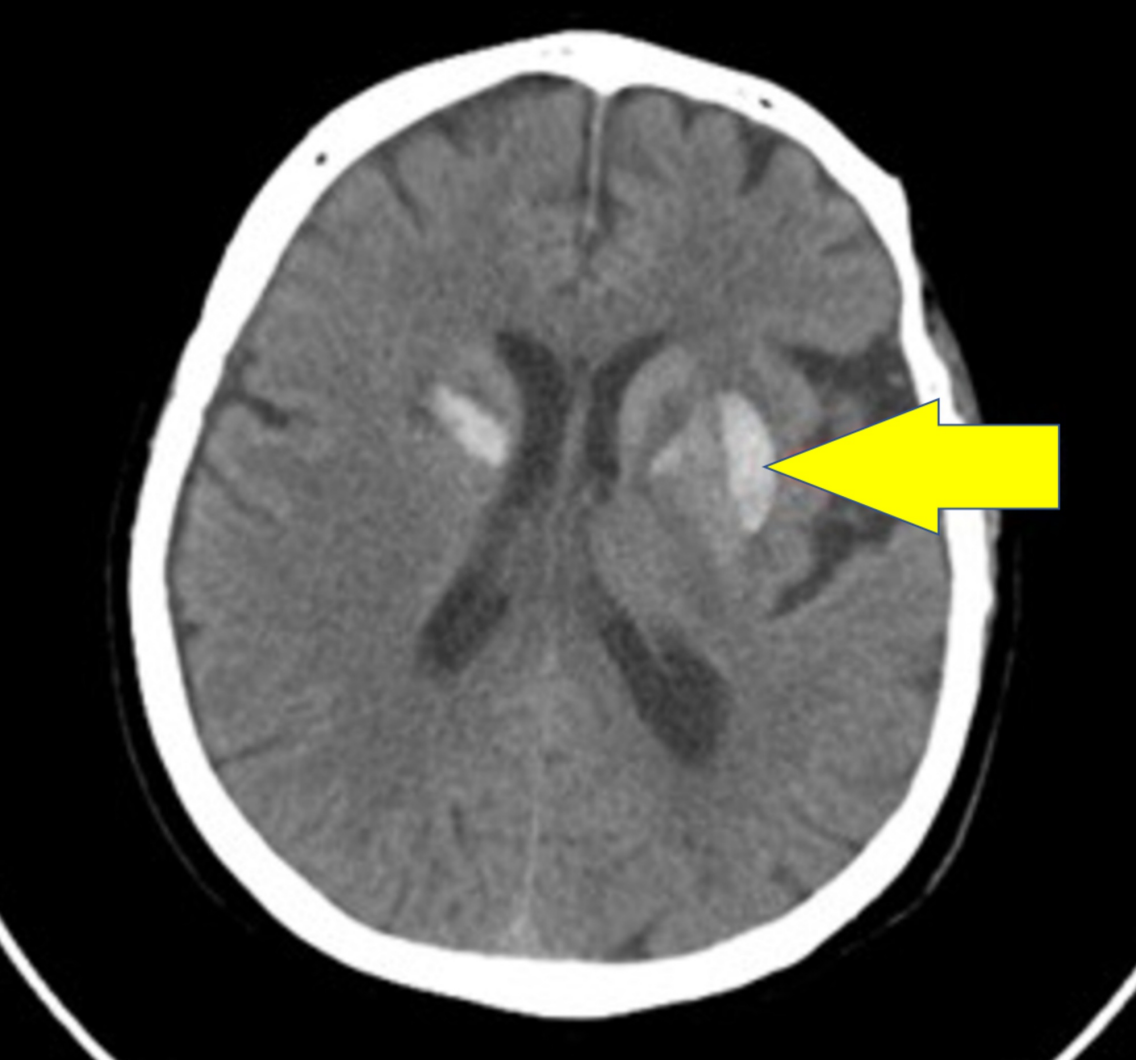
A computed tomography scan of the head that shows acute to subacute changes evident of bilateral basal ganglia hyperdensity matching with a subacute hemorrhagic event
